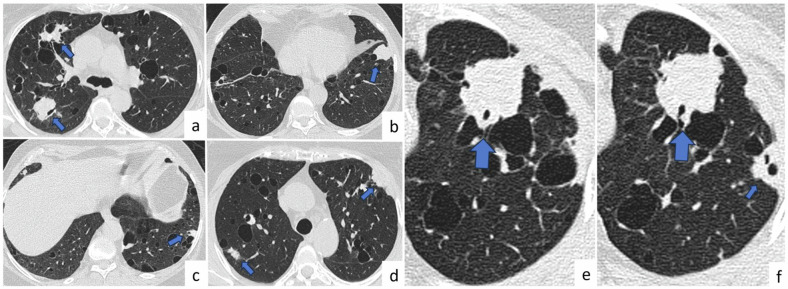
Nodular pulmonary amyloidosis. A 49-year-old female patient with known diagnoses of Sjogren’s syndrome and lymphoid interstitial lung disease. Axial CT images (a–f); show multiple pulmonary nodules in variable sizes and shapes (arrows). There is no significant progression in size of the mass located in the left upper lobe between 2004 (e) and 2008 (f). The diagnosis of amyloidosis was confirmed with CT-guided biopsy
